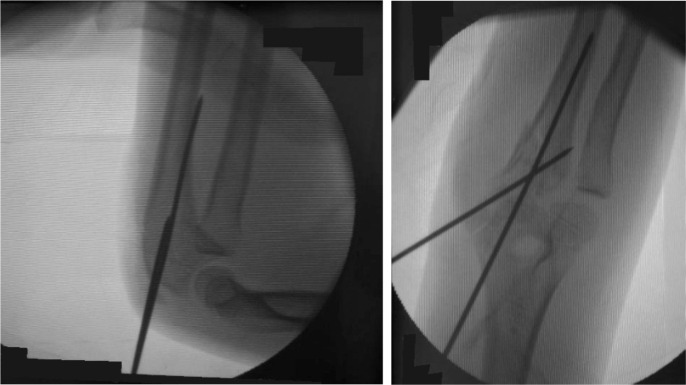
Radiograph of left elbow under image intensifier post--reduction and K-wire insertion.
